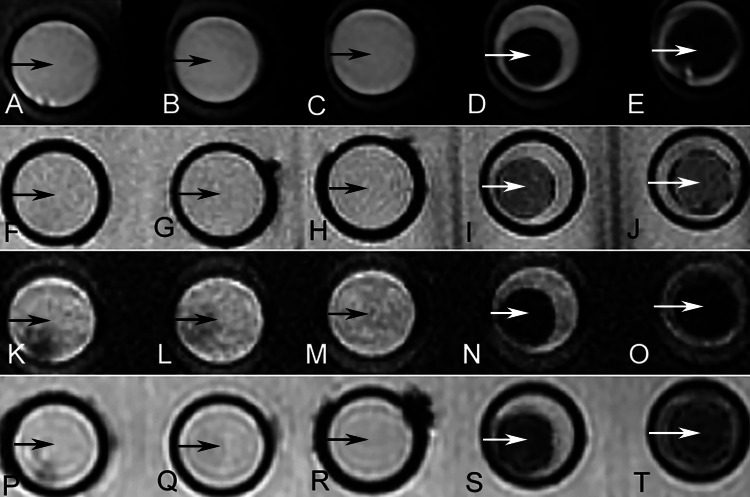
MR signals from other scanning sequences. Images on T2 FSE (from A [5% RBC] to E [95% RBC]), T2 GRE (from F [5% RBC] to J [95% RBC]), FLAIR (from K [5% RBC] to O [95% RBC]), and SWAN (from P [5% RBC] to T [95% RBC]). The lower signal is shown in clots with high RBC content (D, E, I, J, N, O, S, T, white arrows) by comparing with low RBC content (A, B, C, F, G, H, K, L, M, P, Q, R, black arrows). MR: magnetic resonance; FSE: fast spin-echo; RBC: red blood cell; GRE: gradient-echo; FLAIR: fluid-attenuated inversion recovery; SWAN: susceptibility-weighted angiography
